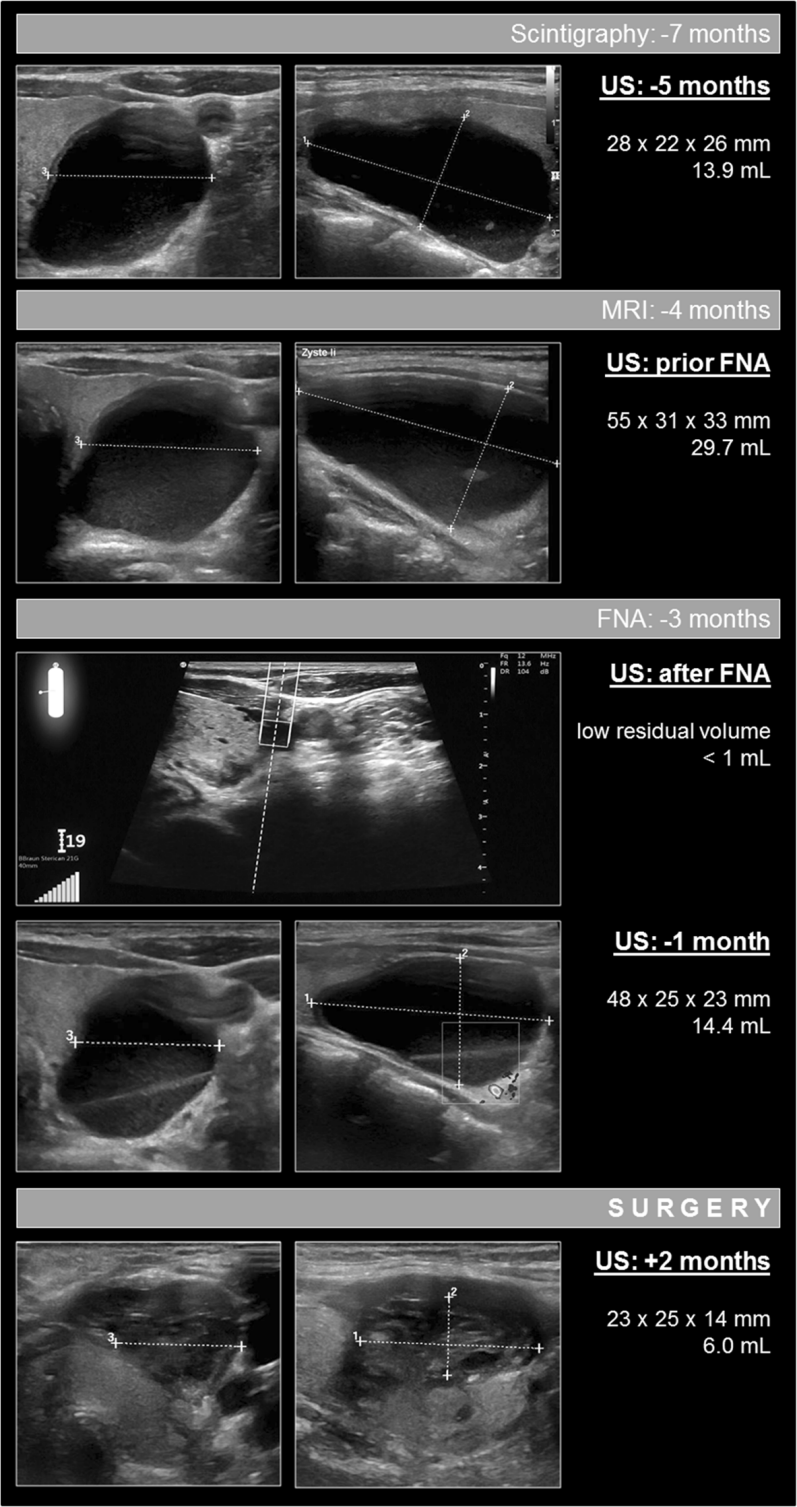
Overview of the diagnostic course. Ultrasonography images of the parathyroid cyst in axial (left image row) and sagittal (right image row) orientations. The last image series shows the post-operative hematoma. Ultrasonography devices used: TOSHIBA Xario, linear probe, 8 MHz; GE LOGIQ S7, linear probe, 8-12 MHz; GE LOGIQ E9, linear probe, 9–15 MHz
